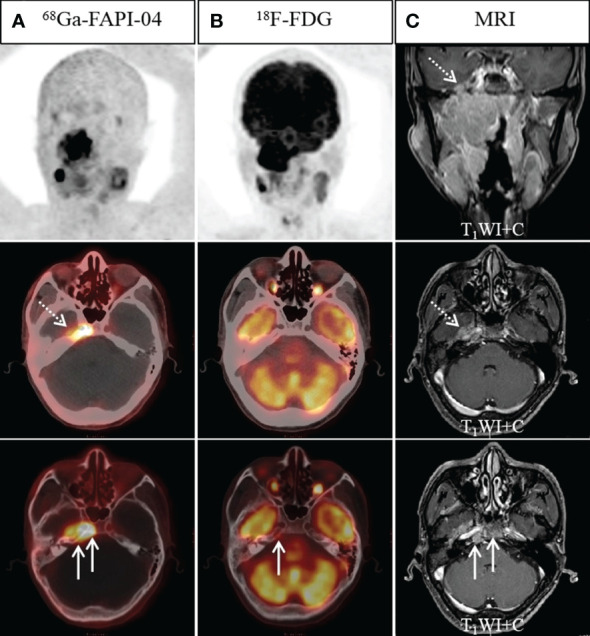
A 45-year-old man with nonkeratinizing differentiated carcinoma. Intense ^68^Ga-FAPI-04 uptake was observed in the left temporal lobe (A, dotted arrow), suggesting intracranial invasion, but ^18^F-FDG PET/CT (B) showed no abnormal intracranial ^18^F-FDG uptake. Moreover, intense ^68^Ga-FAPI-04 uptake was observed in the occipital and right temporal bone (A, solid arrow), while ^18^F-FDG PET/CT only showed low tracer uptake in the right temporal bone (B, solid arrow), which was confirmed by MRI (C, solid arrow). ^68^Ga-FAPI-04 PET/CT revealed more extensive lesions on intracranial and skull base invasion than ^18^F-FDG PET/CT.
